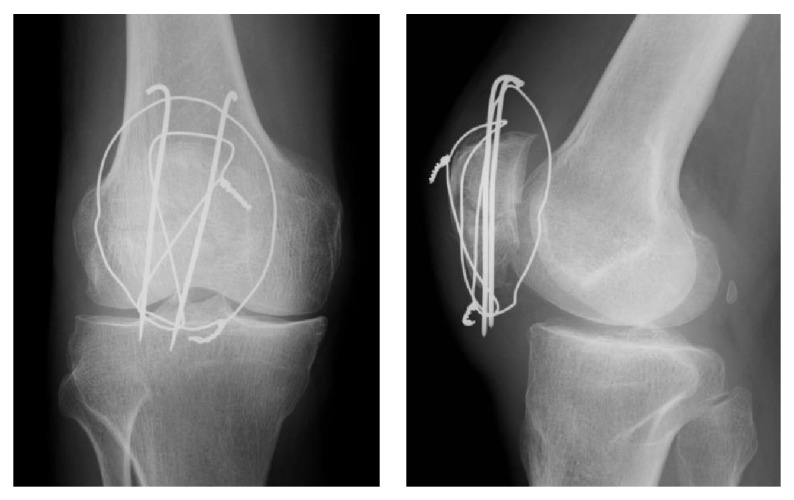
Plain radiographs after the primary surgery.
